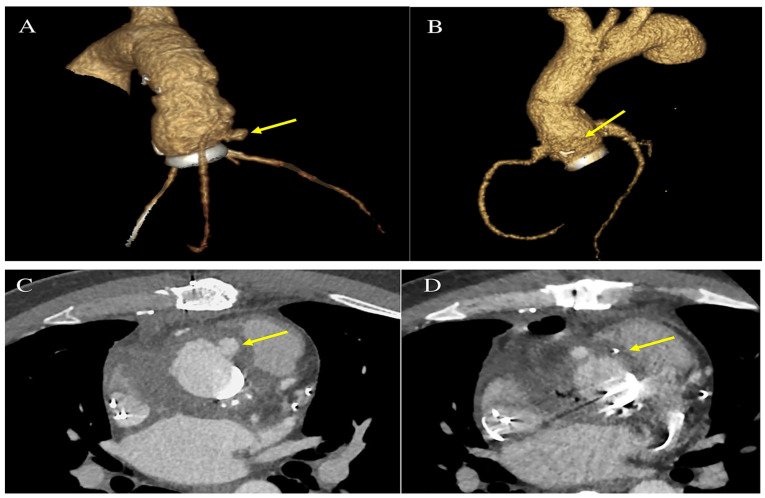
PVE from *E. faecalis*. (A,C) Three-dimensional CT shows a pseudoaneurysm (yellow arrow) before surgery. (B,D) Three-dimensional CT after surgery with complete removal of the pseudoaneurysm (yellow arrow) and replacement of the mechanical prosthesis. Abbreviations: CT, computed tomography; PVE, prosthetic-valve endocarditis.
